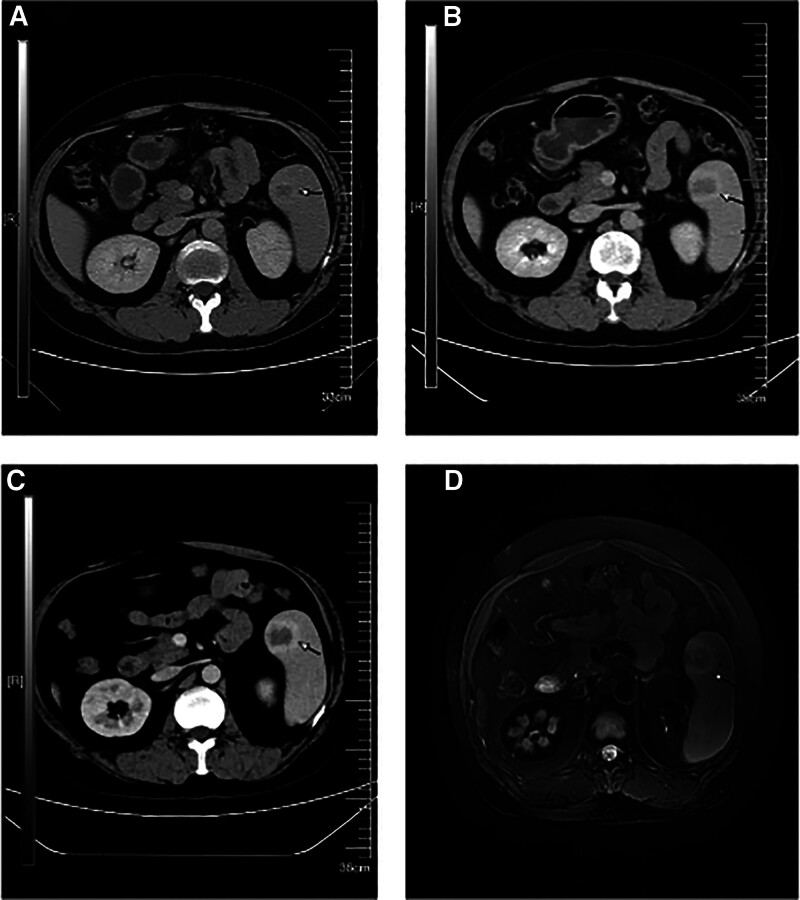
Enhanced CT reveals a localized lesion in the spleen, indicated by the arrow at the lesion site. (A) 2020 Connecticut (B) 2024 Connecticut (C) 2025 CT (D) MRI 2025. CT = computed tomography.
